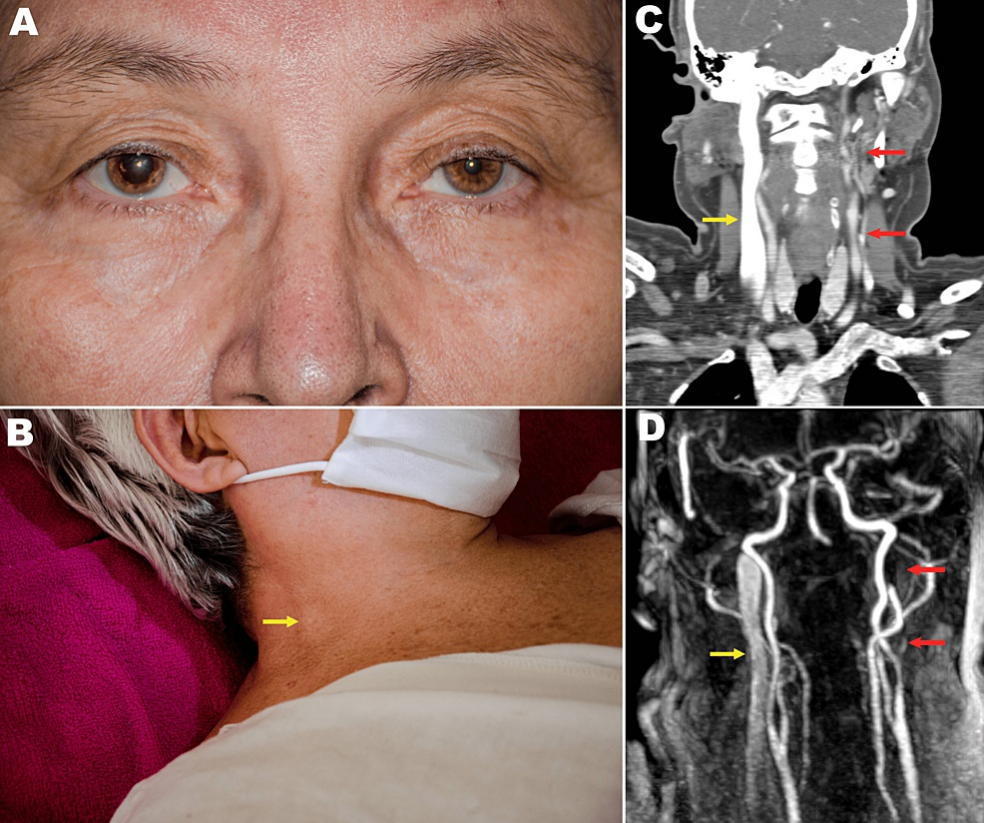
Images of the patient showing Pourfour du Petit syndrome. CT and MR angiography images are also seen. Right mydriasis, exophthalmos, and upper lid retraction (Panel A). Right-sided internal jugular vein distention (yellow arrows) on examination (Panel B), CT angiography (Panel C), and magnetic resonance angiography (Panel D). Red arrows highlight left internal jugular vein agenesis.
